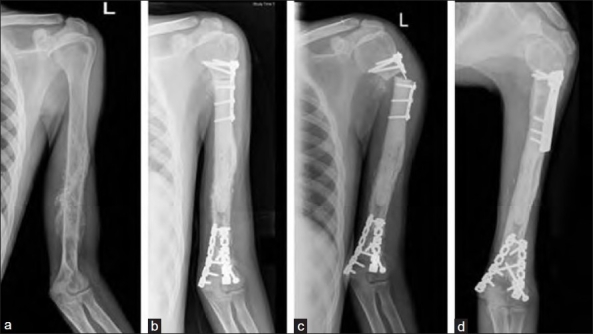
Anteroposterior radiograph of Humerus (a) showing Ewing’s sarcoma (case 8). (b) Postoperative radiograph after extracorporeal irradiation and reimplantation. (c) Non-union and implant failure at proximal diaphyseal osteotomy occured at 10 months followup. (d) Refixation with eventual union at 13 months follow-up radiograph at 30 months shows sound union
